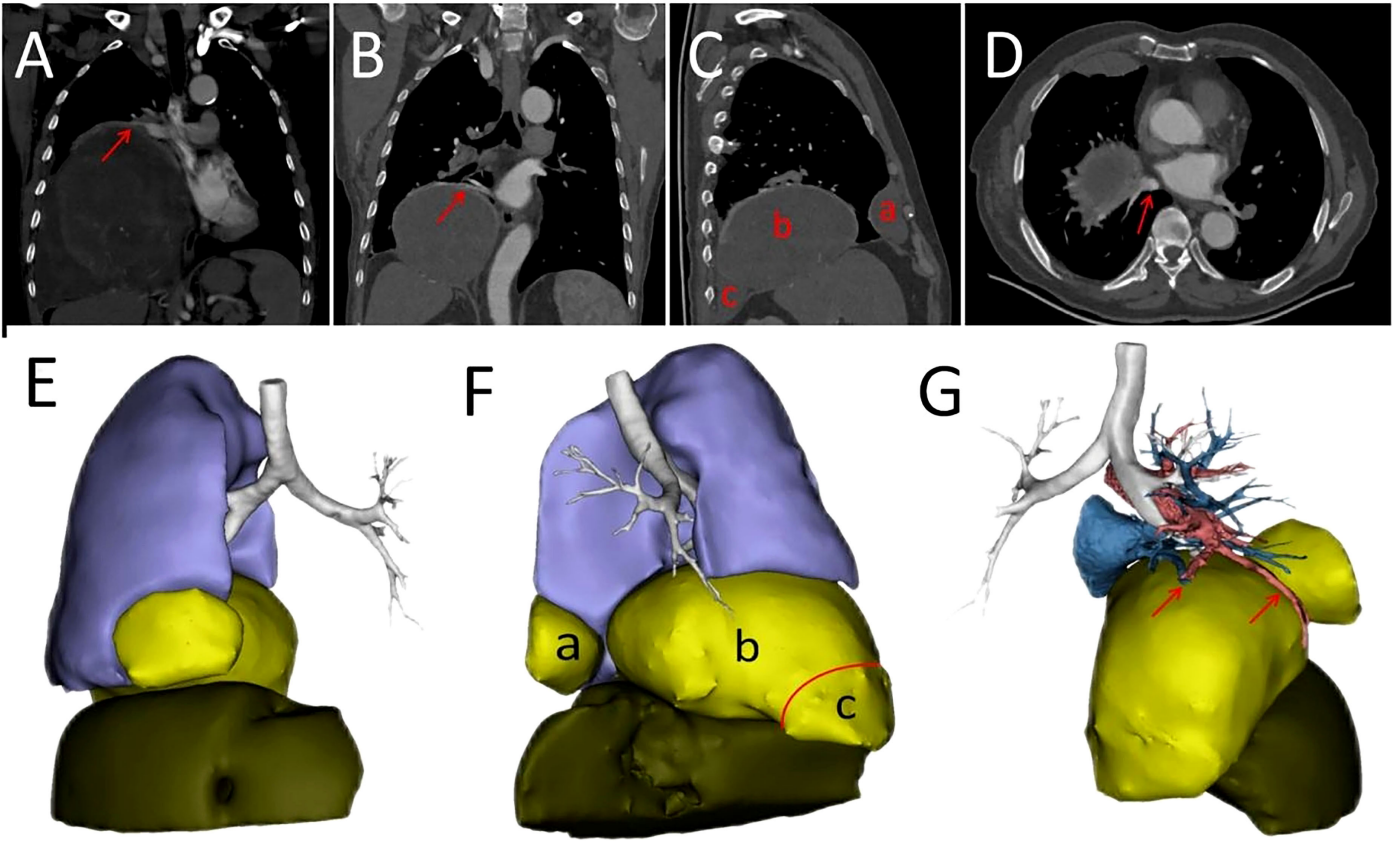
(A) Contrast-enhanced CT scan showing tumor before first surgery of the patient. The arrow represents a rim enhancement, i.e., tumor-feeding vessels around the tumor. (B) Tumor-feeding vessels originated from the inferior pulmonary vein shown by an arrow. (C) The tumor developed recurrence (b) and implant metastases on anterior (a) and posterior (c) pleura. (D) The tumor adjacent to the inferior pulmonary vein as indicated by an arrow. The tumor adjacent to the right lower lobe, right middle lobe of the lung and the diaphragm. (E) Anterior view of a three dimensional (3D) reconstruction. (F) Left view of a 3D reconstruction. Not tight junctions are indicated by the red arc. The tumor originated from posterior pleura (red triangle). Recurrent tumor (b) and implant metastatic tumors (a, c) (G) The relationship between the tumor and the vessels. The blood vessels supplying the recurrent tumor are indicated by arrows.
